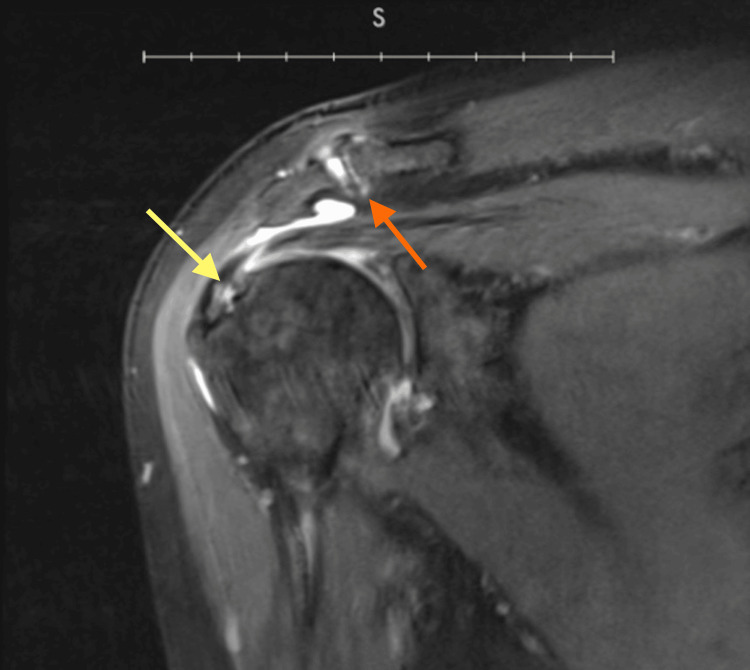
Coronal T1-weighted MRI without contrast Thickening of the supraspinatus tendon and intrasubstance signal consistent with tendinopathy is shown, along with partial-thickness articular surface tear of the supraspinatus and delamination of tendon fibers (yellow arrow). Subacromial bone spur and hypertrophic changes are seen (orange arrow).
